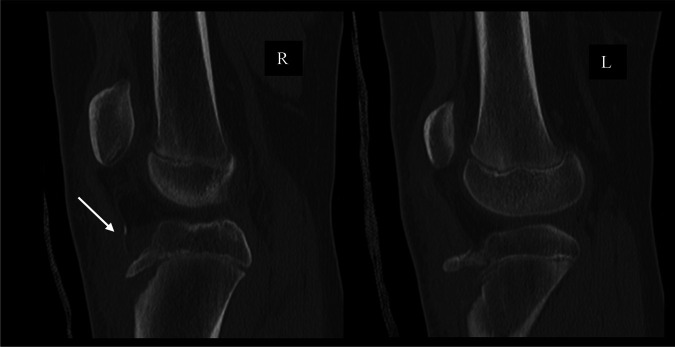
CT scan of bilateral knees did not reveal any intra-articular involvement of the injury. The tibial tubercle sleeve is better appreciated on this sagittal cut of the right knee (arrow).
